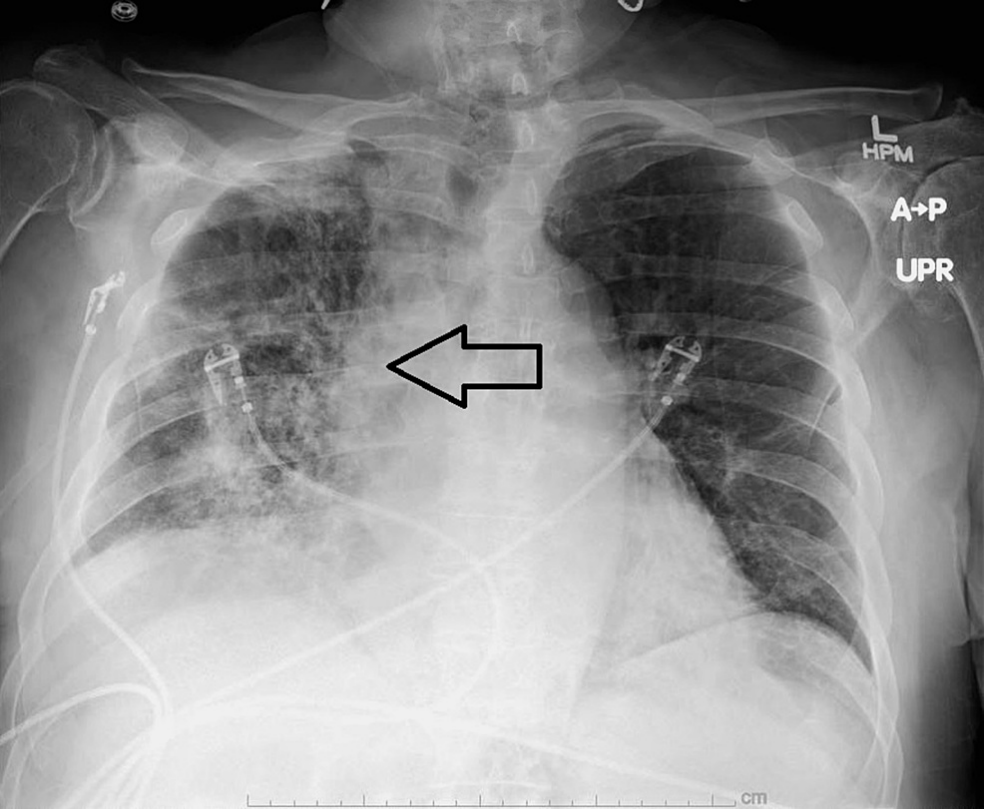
Our patient's admission AP chest radiograph showing unilateral, right-sided infiltrates AP: anteroposterior
